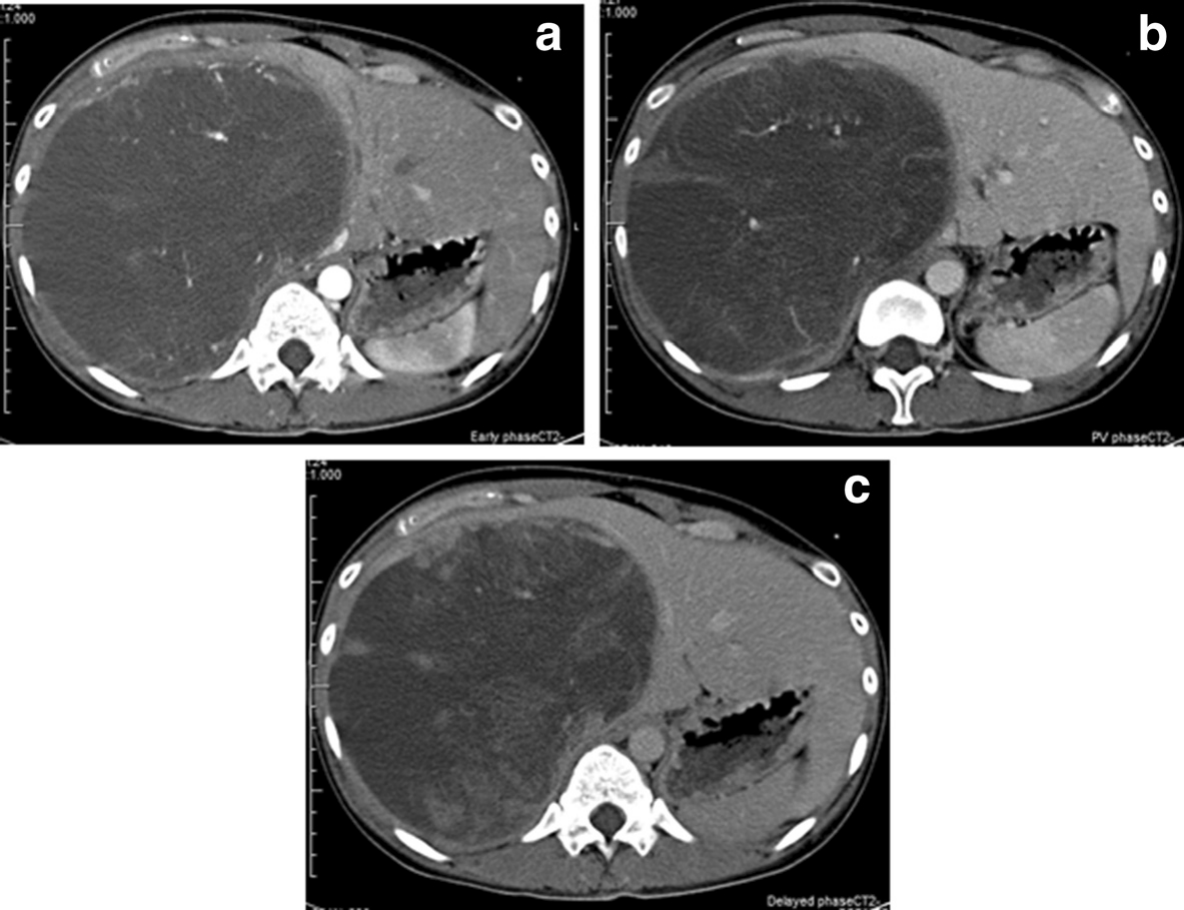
(a, b, and c) Abdominal computed tomography scan showing a well-defined, low-density large mass (21 × 19 × 14 cm) with heterogeneity in the right segments of the liver. This tumor had vessels, septa, no enhanced-effect, and no solid compartment (a, early phase; b, portal vein phase; and c, delayed phase).
